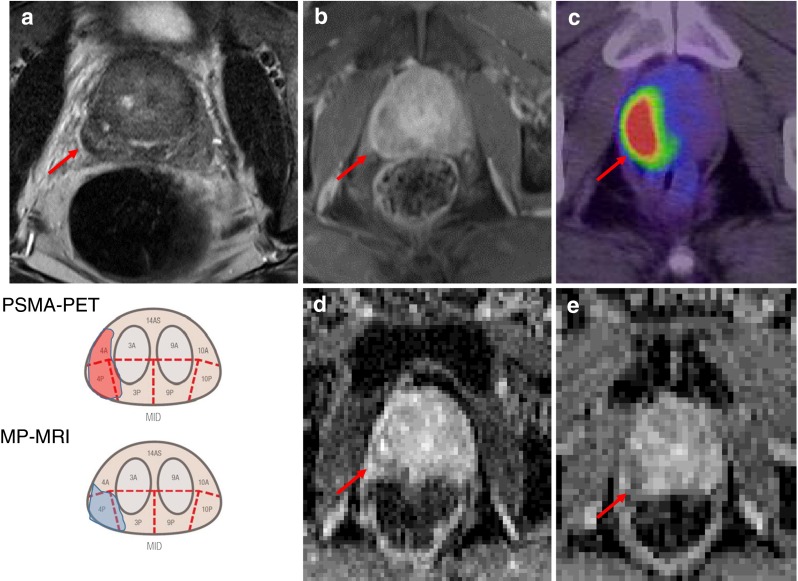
Example for a near-total agreement. Biopsy proven prostate cancer in the right, peripheral zone. In MP-MRI, a signal drop in T2w (a), fast wash-out in contrast enhanced T1w sequence (b), focal diffusion restrictions in DWI (d) and corresponding ADC-map (e) were interpreted as tumor infiltration of segment 4P. On a visual basis, the PSMA-PET (c) presents a very similar tumor extent, but was scored as tumor spread into the segments 4A and 4P
